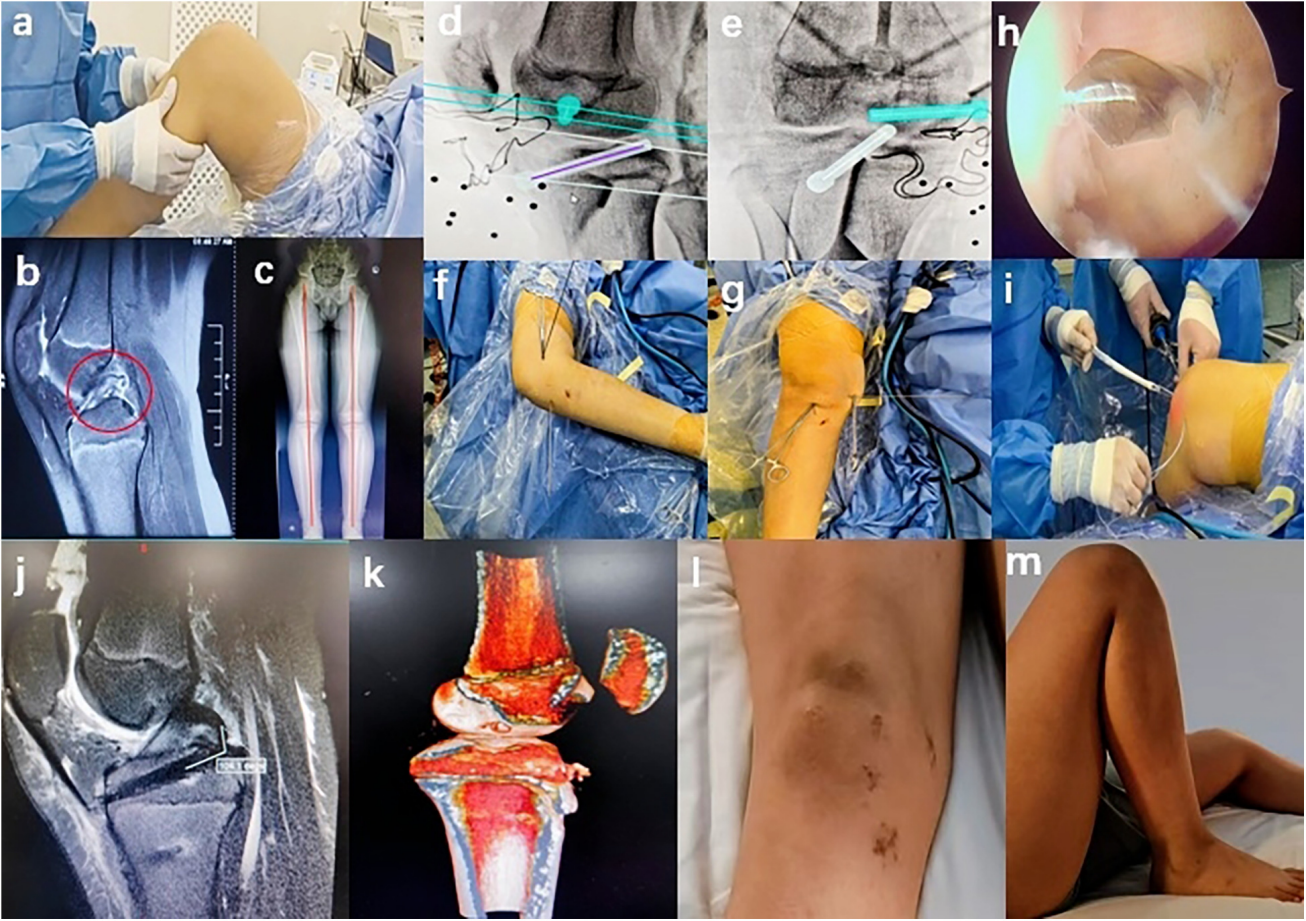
Case presentation: (a) positive posterior drawer test, (b) preoperative MRI of the right knee revealing discontinuity in the middle and lower segments of the PCL with partial retraction of the ruptured ends, (c) preoperative full-length x-ray of both lower extremities indicating no significant abnormalities in their alignment, (d,e) blue and white shapes representing the femoral and tibial bone tunnels, respectively, (f,g) guide pin positioning, (h) arthroscopic observation, (i) tendon pulling, (j) postoperative MRI showing that the angle between the graft and the tibial tunnel was approximately 104.1°, (k) postoperative CT showing that both the femoral and tibial bone tunnels were within the growth plate, (l) surgical incision, and (m) knee flexion to full angle achieved at week 8.
